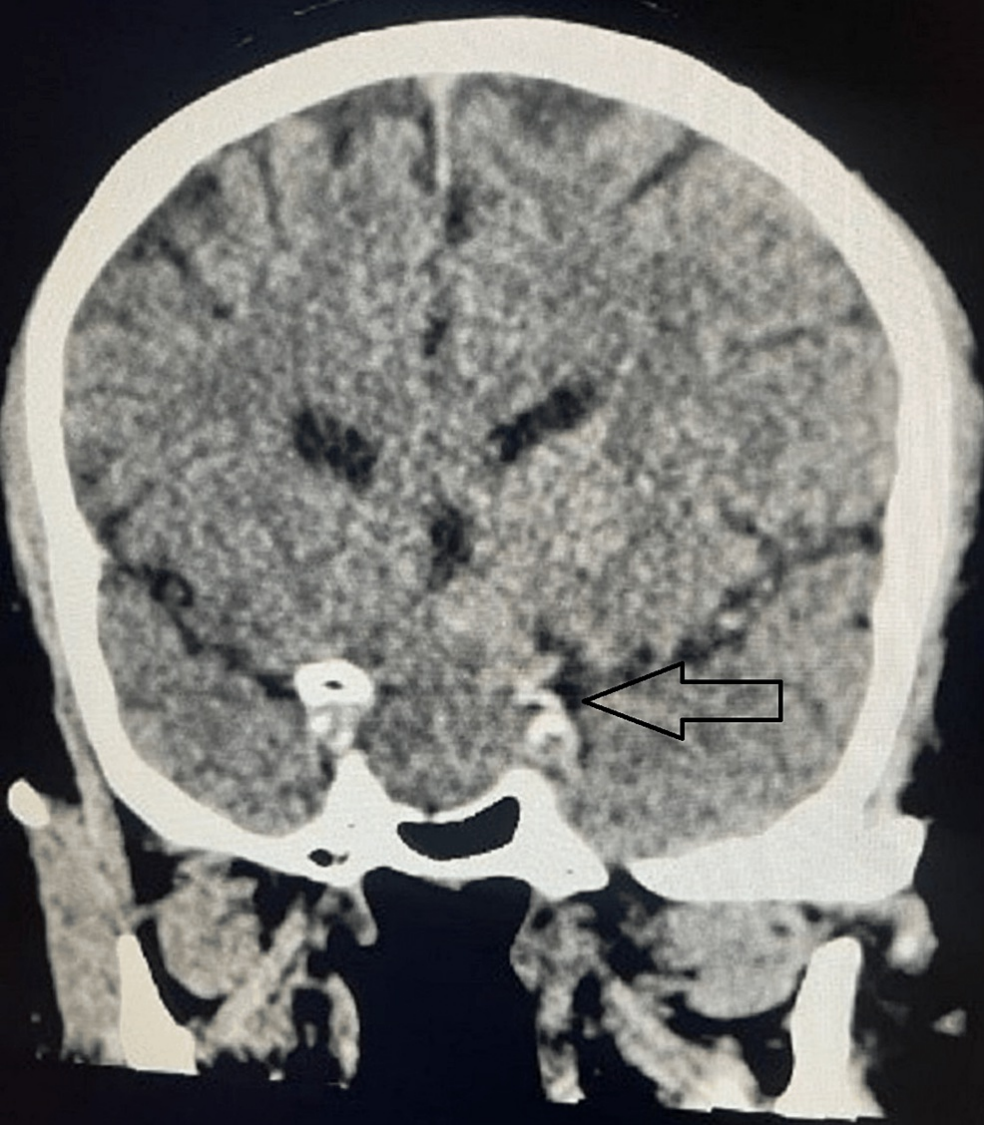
CT scan coronal view showing sellar and suprasellar mass lesions.
